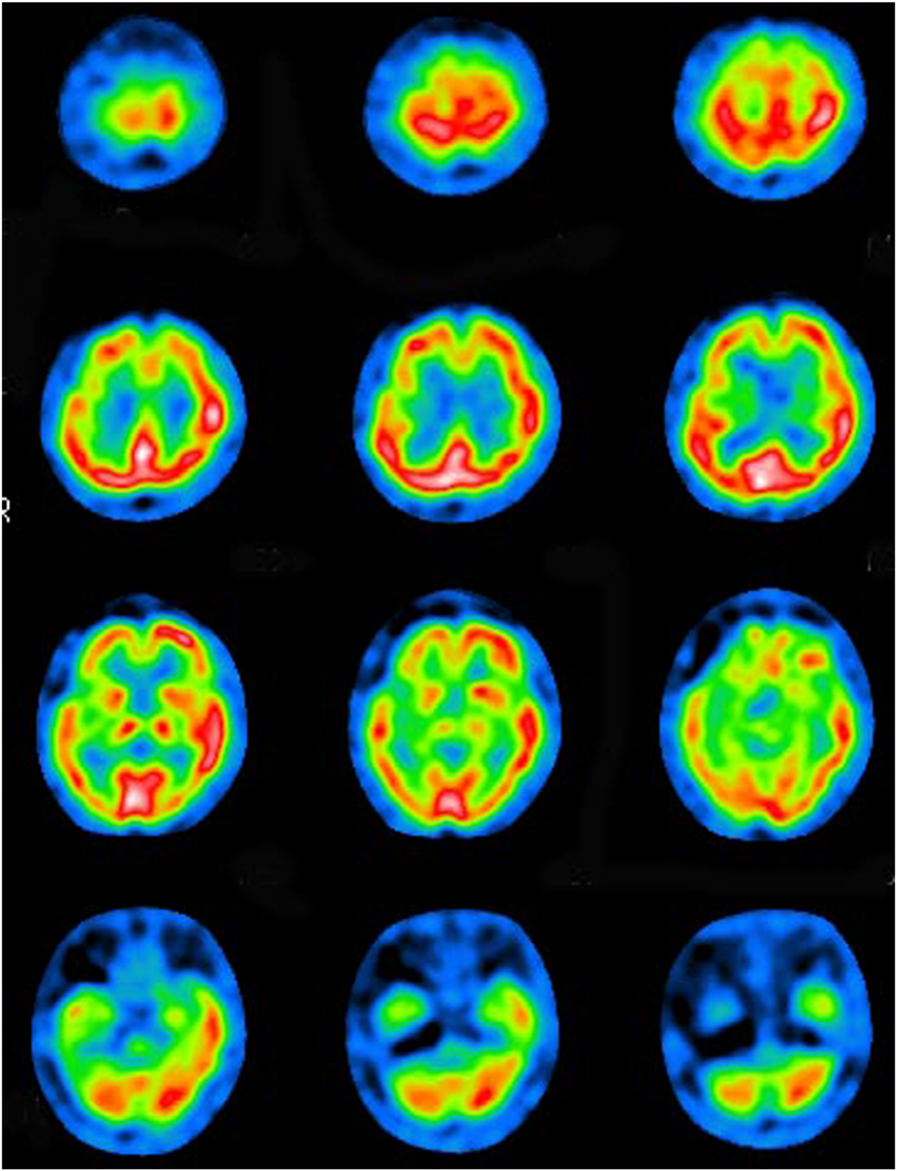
Single photon emission computed tomography imaging revealed obvious hypoperfusion of the right frontal and temporal cortices, basal ganglia and brainstem, while perfusion of right cerebellar cortex was mildly impaired.
